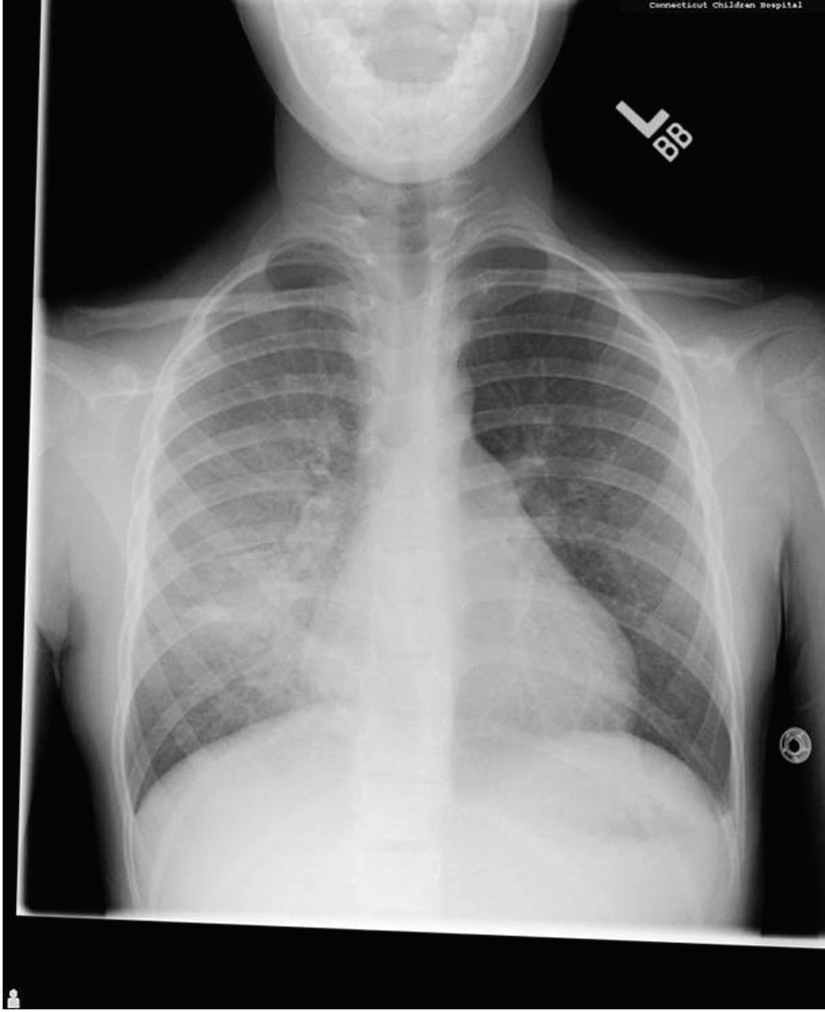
Initial chest X-ray demonstrated a diffuse airspace filling process throughout the right lung and a small airspace opacity in the left upper lobe.
